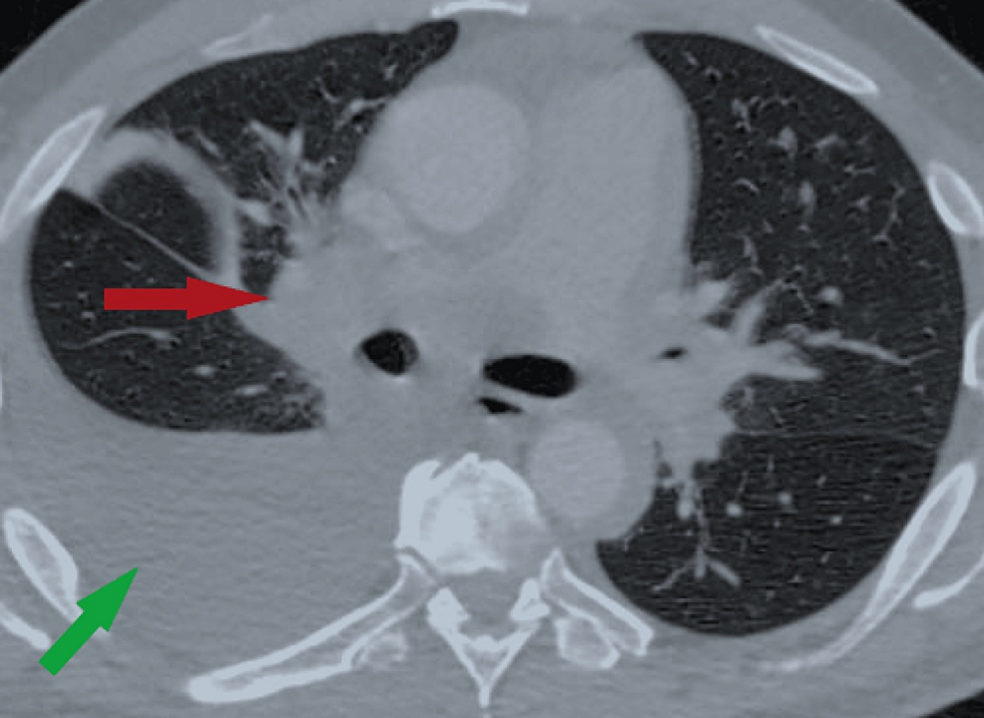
Contrast-enhanced CT scan of the thorax. The green arrow points to a very large right pleural effusion occupying most of the right pleural space. There is no significant left pleural effusion. The red arrow points to a large right perihilar mass with some irregularity.
